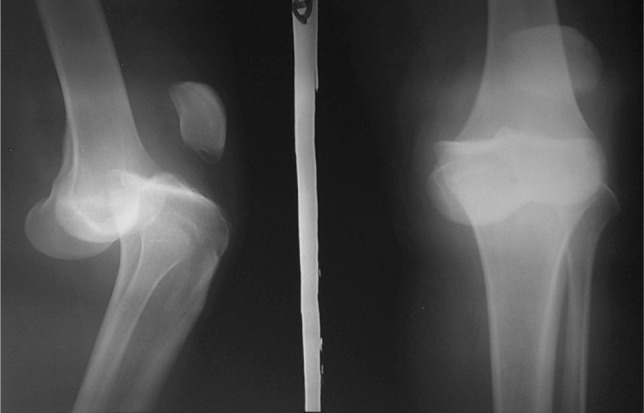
Radiographs of knee joint in antero-posterior and lateral views showing dislocated knee joint.
